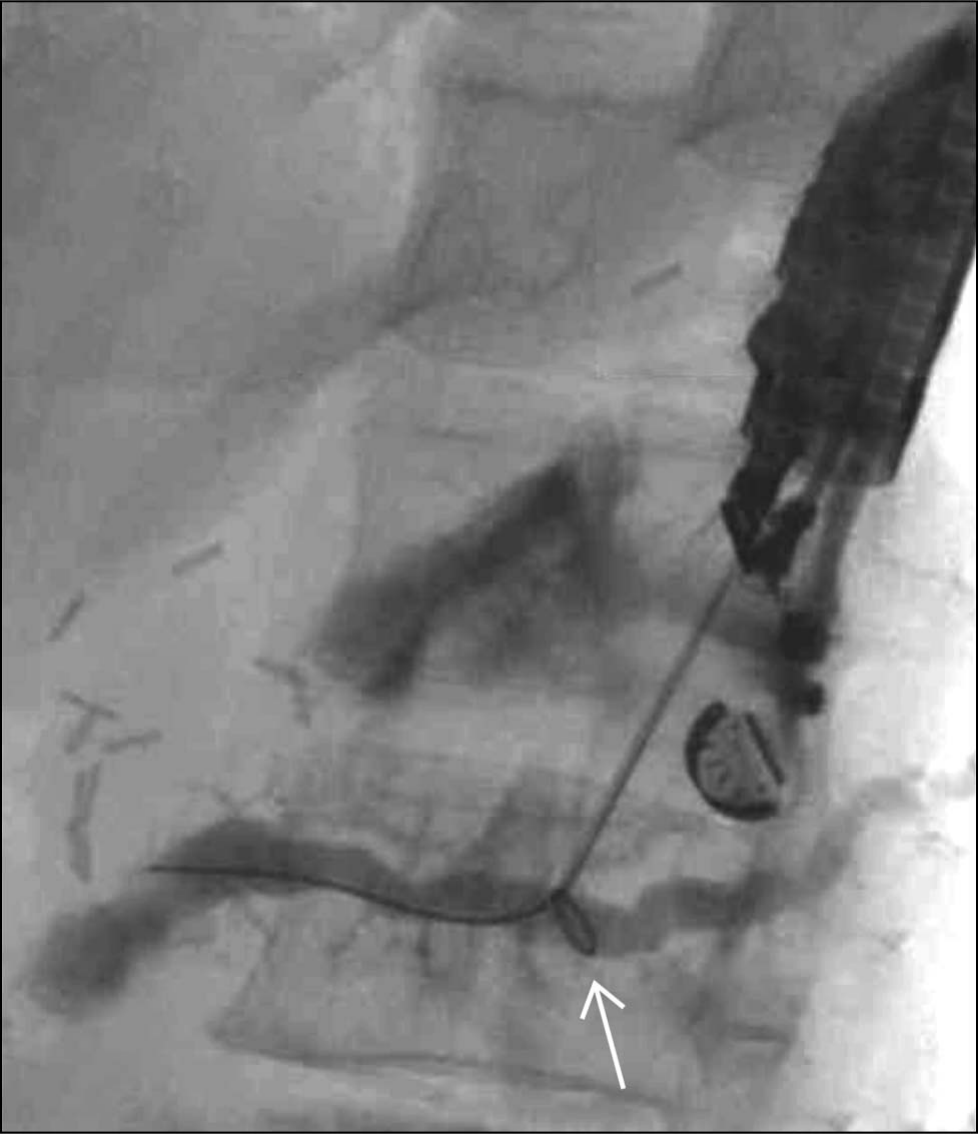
Wire manipulation after endoscopic ultrasound-guided puncture of the main pancreas duct resulted in formation of an alpha loop within the main pancreas duct (arrow).
